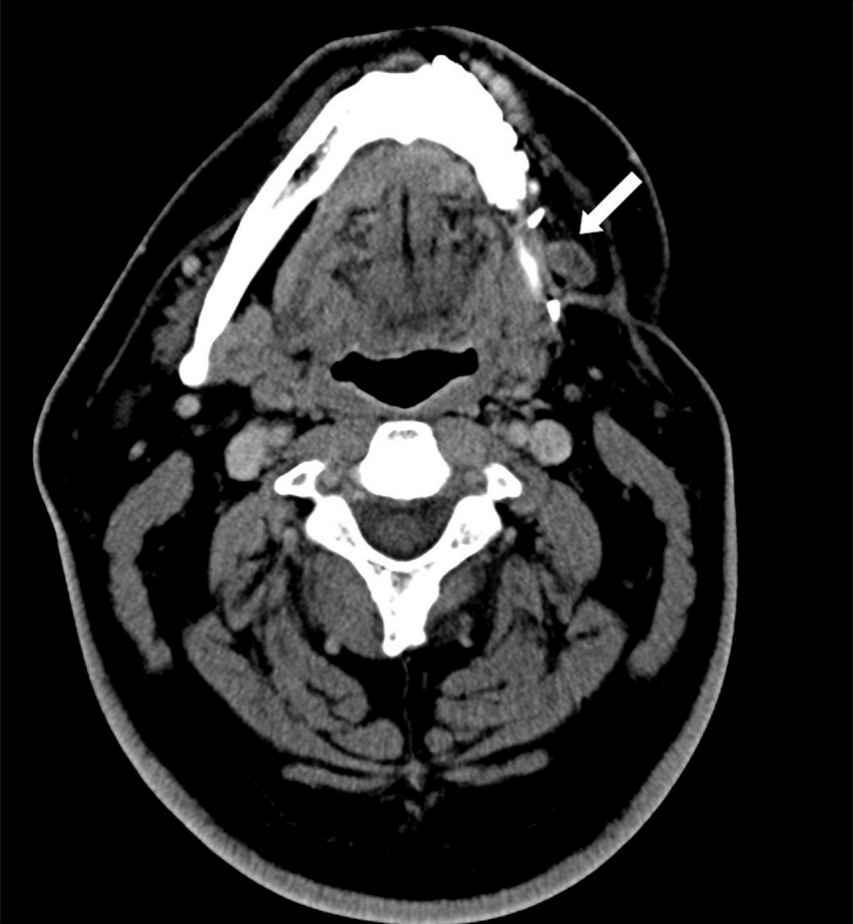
Axial CT scan showing a large pathological submandibular lymph node with heterogeneous internal density (white arrow).
